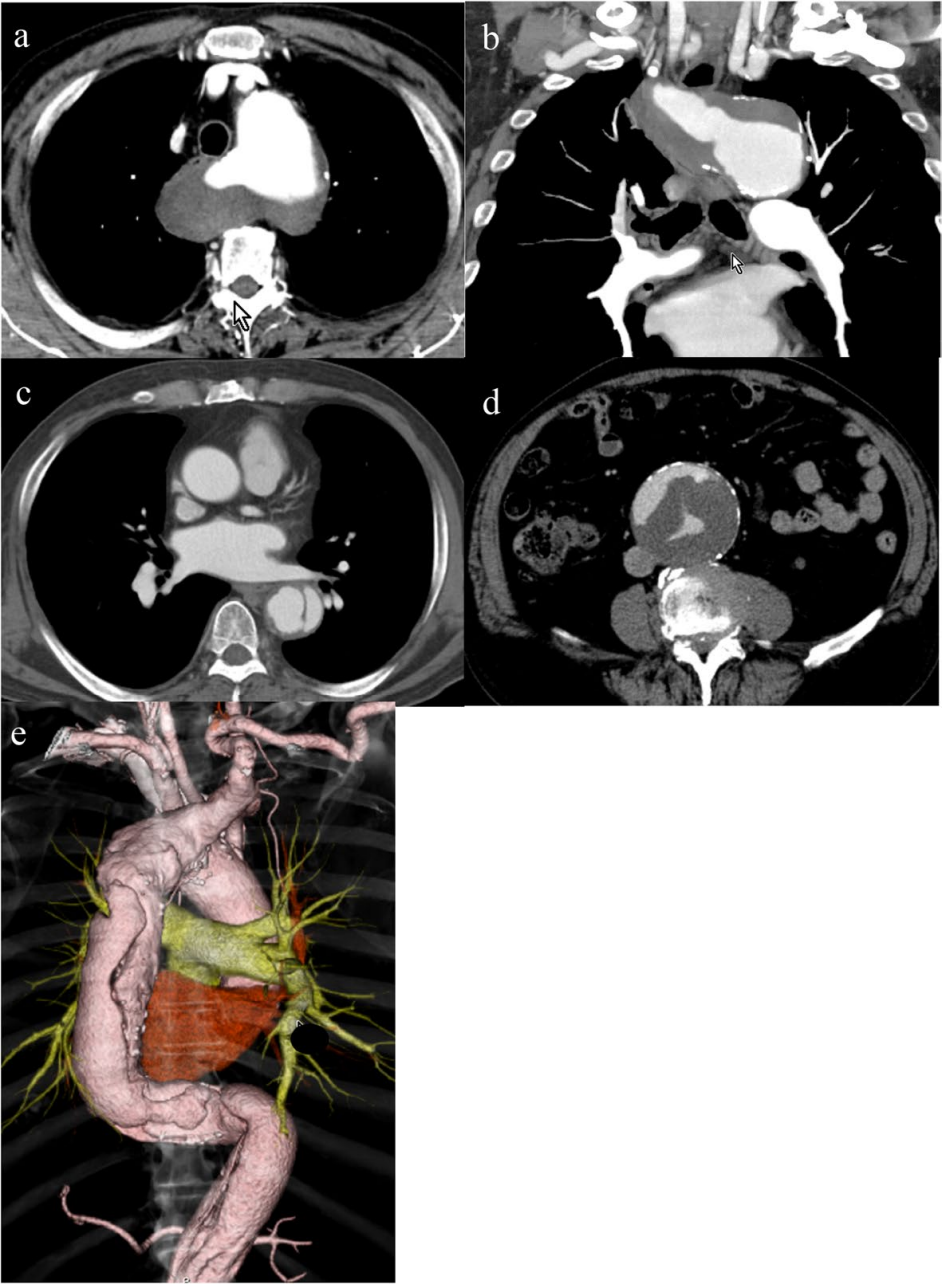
Enhanced computed tomography findings. a, b The aberrant right subclavian artery branched from the descending thoracic artery and its origin was dissected and dilated. c Aortic dissection of partial thrombosis type in the descending thoracic aorta. d Aortic dissection involving an abdominal aortic aneurysm. e 3D computed tomography reconstruction shows the aberrant right subclavian artery running distal to the left subclavian artery
